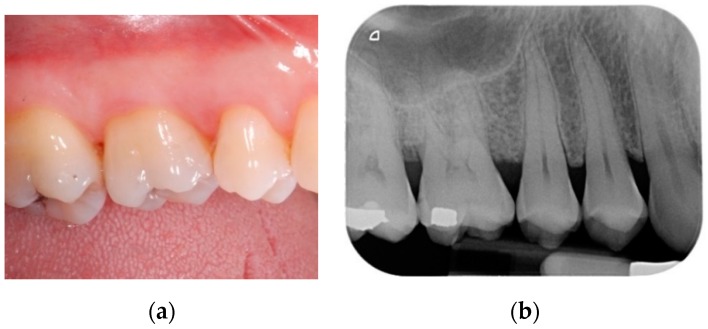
(a) Clinical view of the treated area at 12-month follow-up; (b) periapical radiograph at 12-month follow-up.
